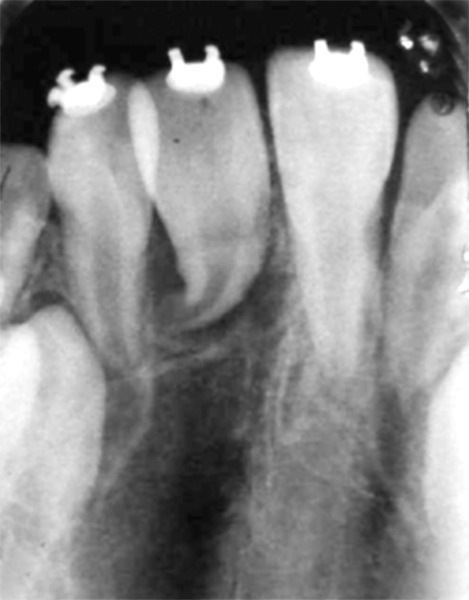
Postoperative IOPA radiograph
